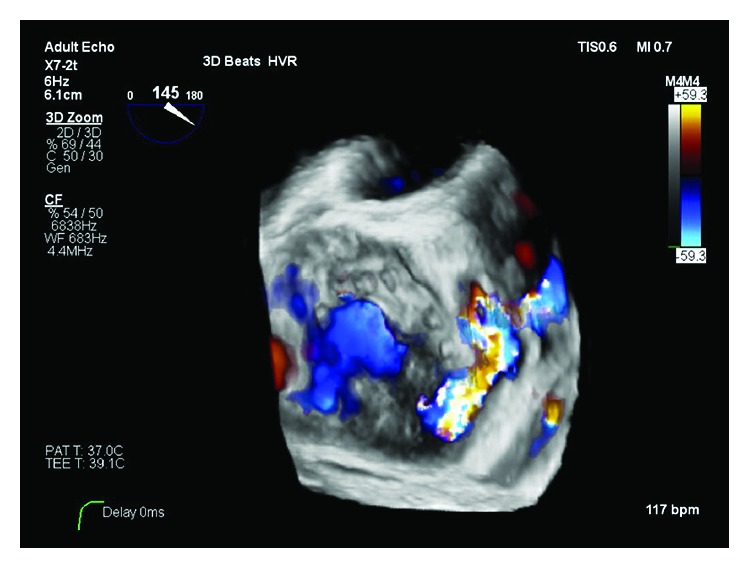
TEE 3D reconstruction with color Doppler showing a bioprosthetic mitral valve with paravalvular leak on the short axis.
